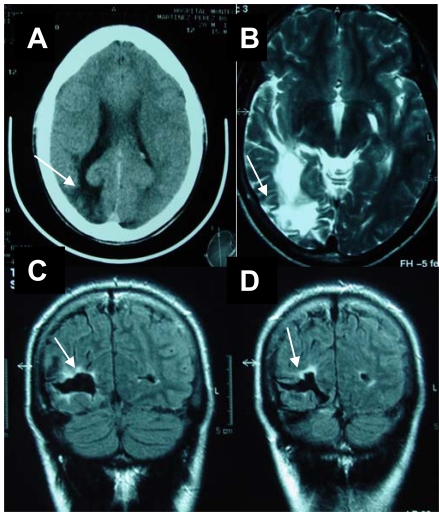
a. Brain CT. Hypodense right occipital lobe lesion (arrow) with dilatation of the ventricular horn and widened cortical sulci (atrophy). b. Brain MRI (FLAIR). Axial section. Hyperintense white matter signal in the occipital lobe (arrow), ventricular dilatation and wid-ened sulci. c-d. Brain MRI (T2 weighted). Coronal section. Hypointense signal in the right occipital lobe with a peripheral hyperintensity (arrow) suggestive of a chronic ischemic infarct.
